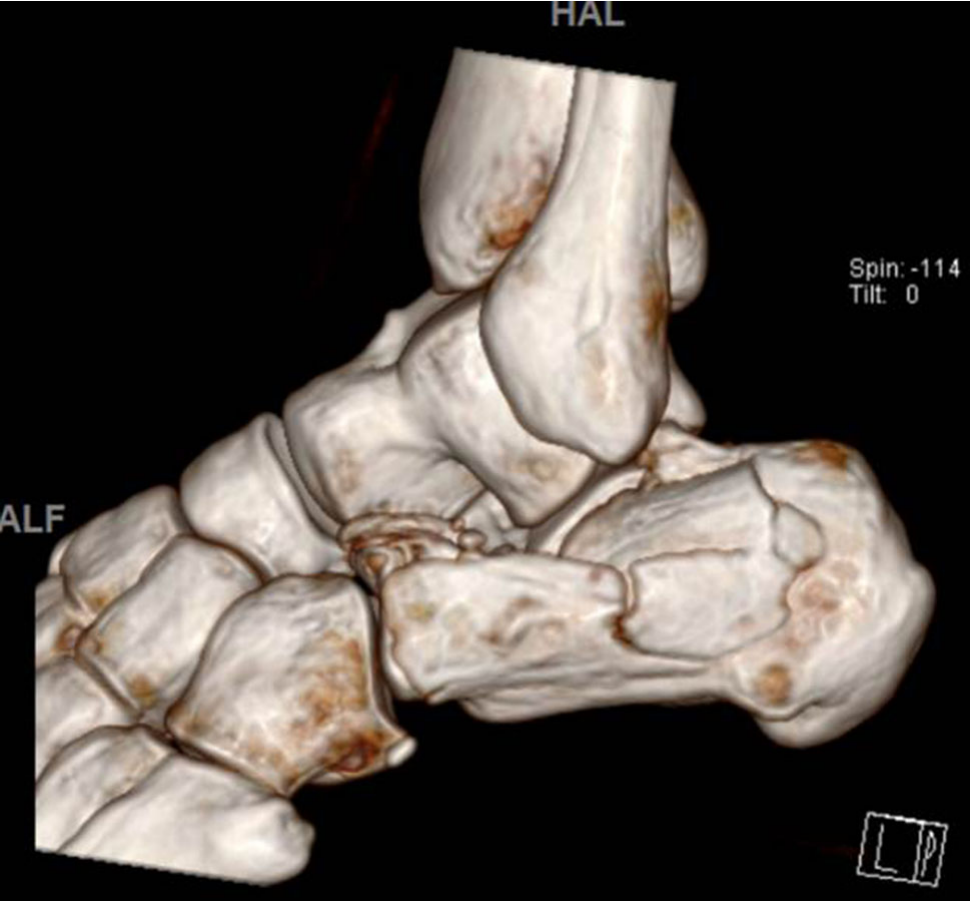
CT reconstruction Sanders II, joint split. Constant fragment medially, lateral articular piece rotated by 90°
